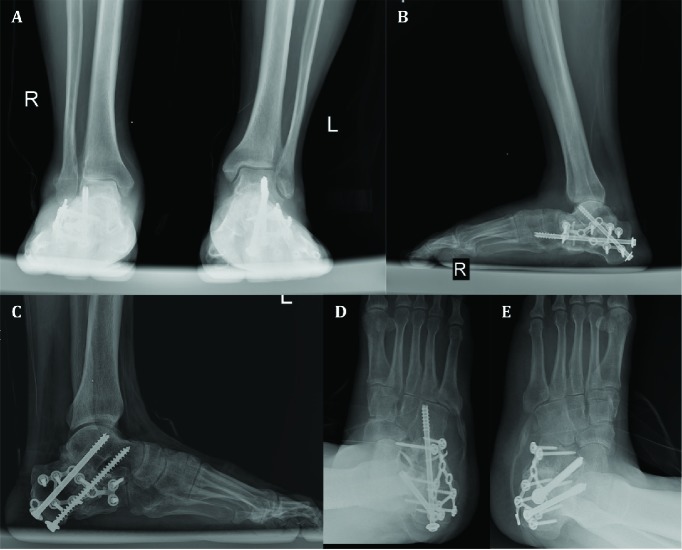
At 14-month postoperative follow-up, anteroposterior ankles (A), lateral ankles (B, C), and oblique feet (D, E) radiographs demonstrating bony union
